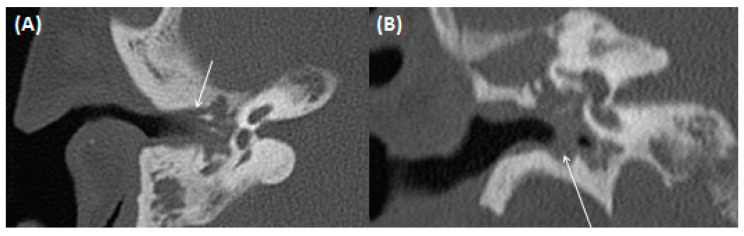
CT of an 84-year-old female patient with axial (A) and coronal (B) bone window images of the right middle ear. Complete obstruction of the middle ear including the epi-, meso-, and hypotympanum is shown. Images also show a swollen EAC, and the eardrum cannot be clearly defined. Only faint delineation of the auditory ossicles is observed. Bony thinning from the cortex to the base of the skull without clear interruption is present as well.
